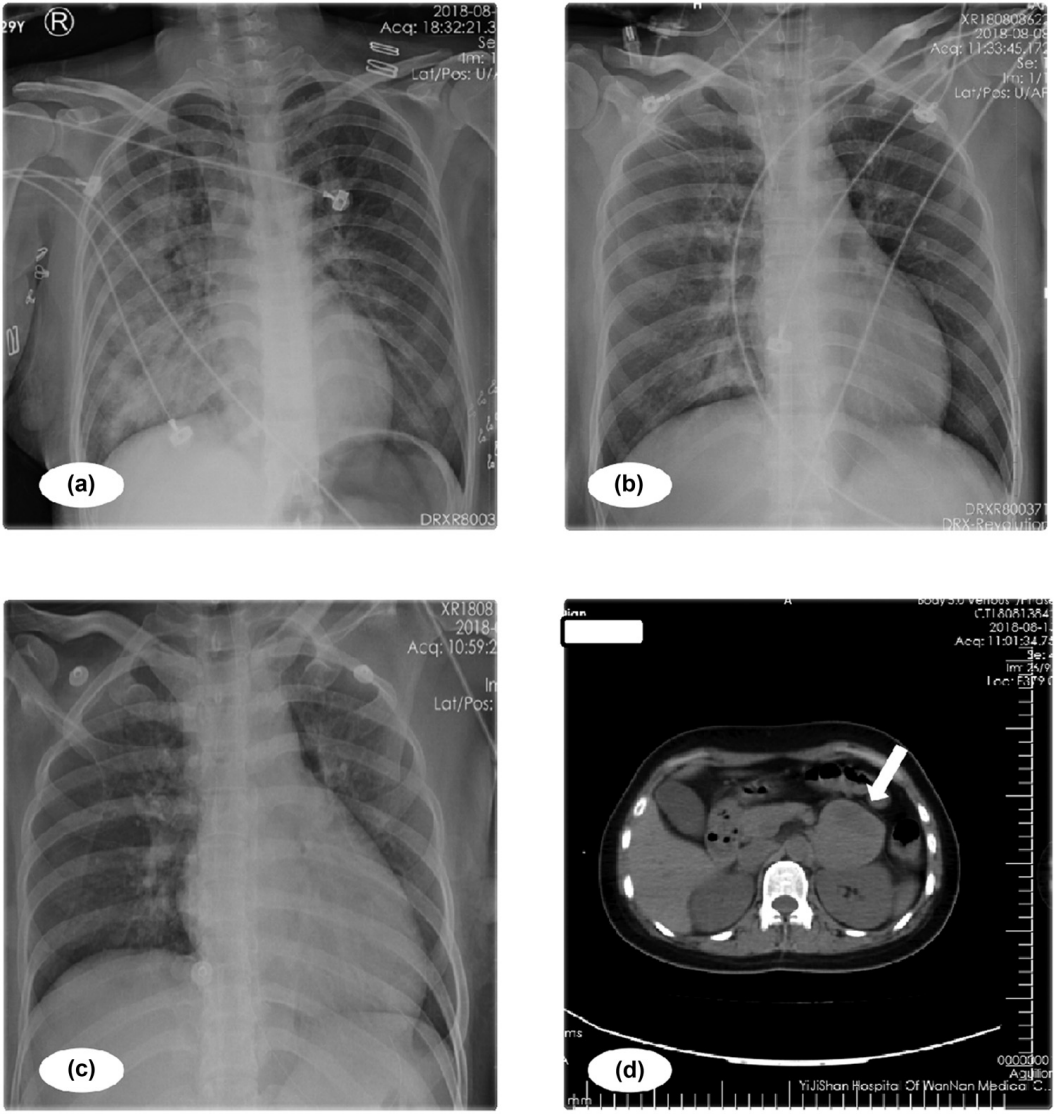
Radiologic examination of the patient. (a) Chest X-ray showing serious interstitial and alveolar oedema. (b and c) Chest posteroanterior shows interstitial and alveolar oedema improved. (d) CT revealed a left suprarenal tumour of 5.8 cm × 5.7 cm in size with necrosis (white arrow).
